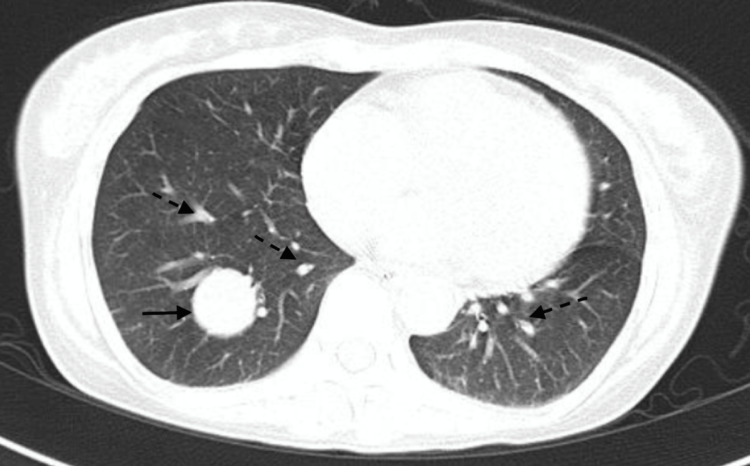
CT thorax Showing metastatic nodule (solid arrow) in the right lower lobe with multiple suspicious nodules (broken arrows) in both lungs
